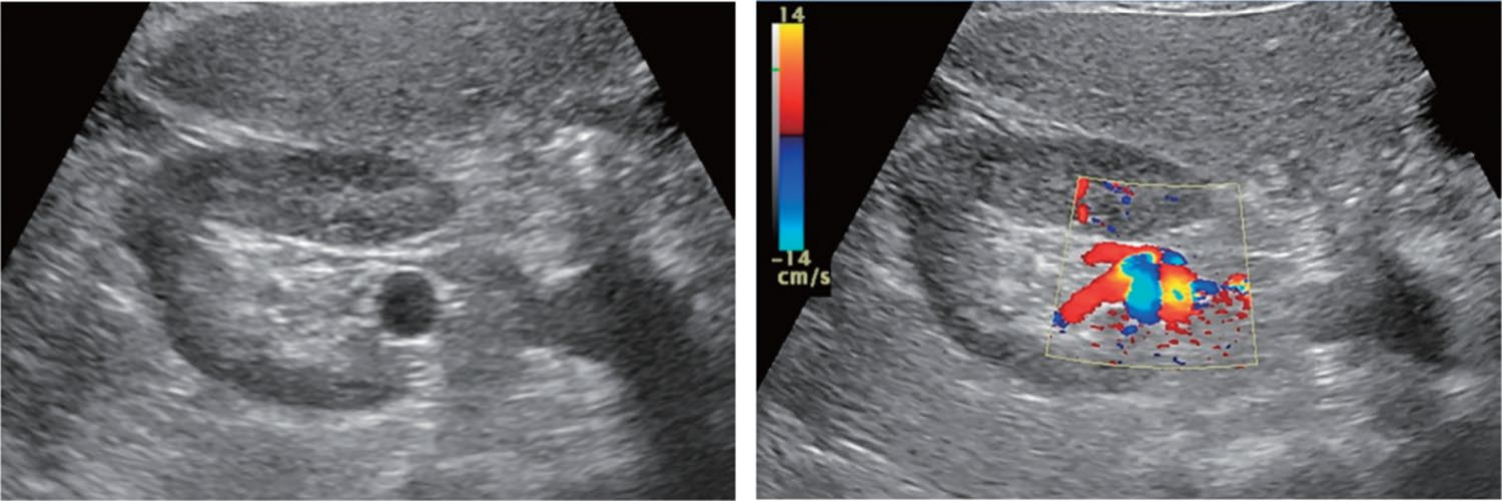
Other findings. Vascular abnormality. A cystic lesion is present in the renal hilus (left), and a blood flow signal is present on color Doppler (right). Left: renal short-axis view b-mode; right: color Doppler. Category 2, Assessment D2
